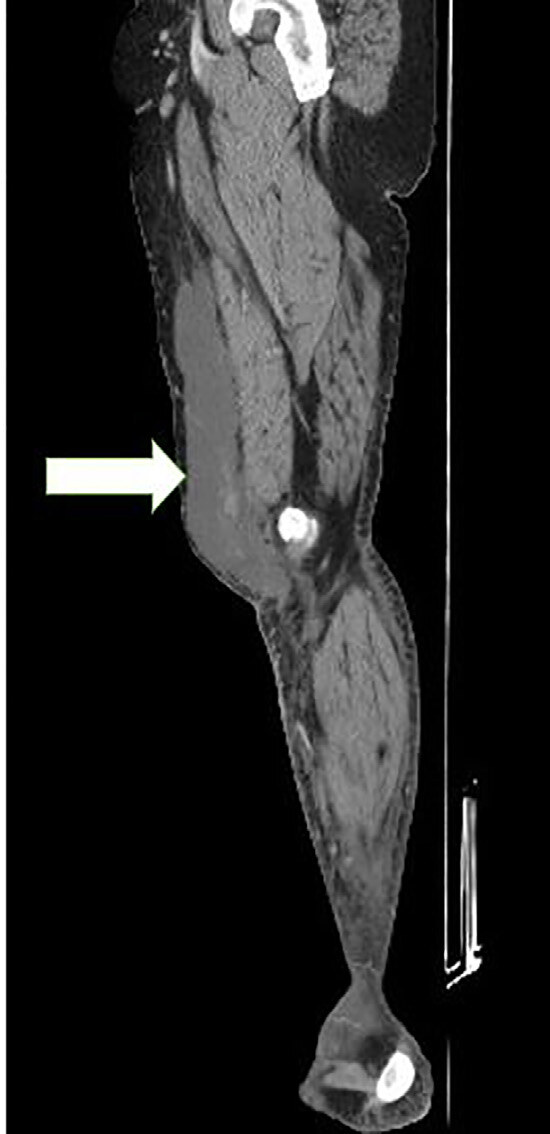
Computed tomography of the right lower extremity showing an internal hemorrhage and internal degloving injury of the thigh (arrow).
